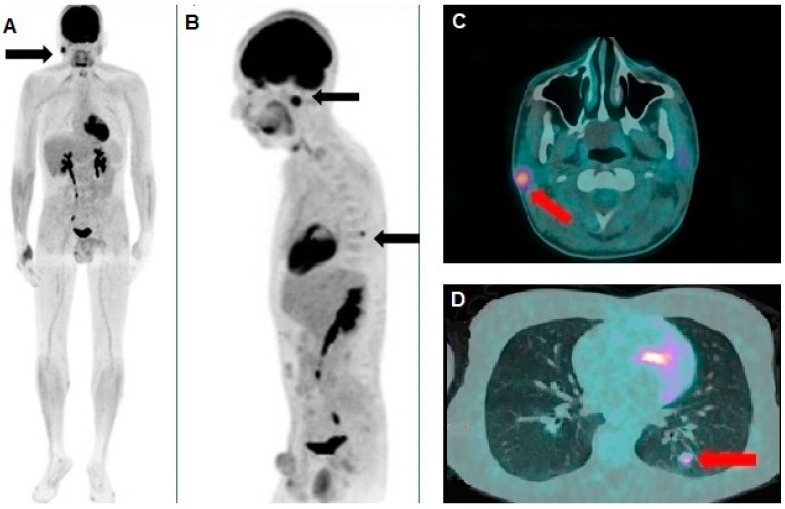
(A) Whole-body [^18^F]FDG PET/CT revealed significant hypermetabolism in the right parotid gland, as indicated by the black arrow, suggesting a metabolically active lesion. (B) Oblique sagittal reconstruction showing pathological [^18^F]FDG uptake both in the parotid gland and in an additional focal area in the left hemithorax, compatible with a pulmonary nodule (black arrows). PET images were assessed using both qualitative and quantitative analyses. Specifically, any lesion demonstrating tracer uptake higher than the surrounding background and not attributable to physiological activity was considered potentially pathological. Using dedicated software (PET VCAR, version 4.7, GE Healthcare, WI, USA), the maximum standardized uptake value (SUVmax) was calculated. Additionally, each lesion was segmented by applying a threshold of 42% of the SUVmax, and the metabolic tumor volume was automatically measured. The axial PET/CT fusion images of the skull (C) provided further characterization of the lesions, showing hypermetabolism in a small nodular formation within the right parotid gland (red arrow), with a maximum standardized uptake value (SUVmax) of 6.9 and a metabolic tumor volume (MTV) of approximately 3 cubic centimeters (cm^3^). Additionally, (D) the known pulmonary nodule in the apical segment of the left lower lobe (red arrow), previously identified on chest CT, exhibited an SUVmax of 4.8 and an MTV of about 0.5 cm^3^. Considering the patient’s clinical history, both lesions were interpreted as metastatic disease from the XP-associated melanoma. Consequently, the patient started anti-programmed cell death protein 1 (anti-PD-1) therapy with nivolumab at a dose of 480 mg every four weeks, which was well tolerated without significant adverse effects.
